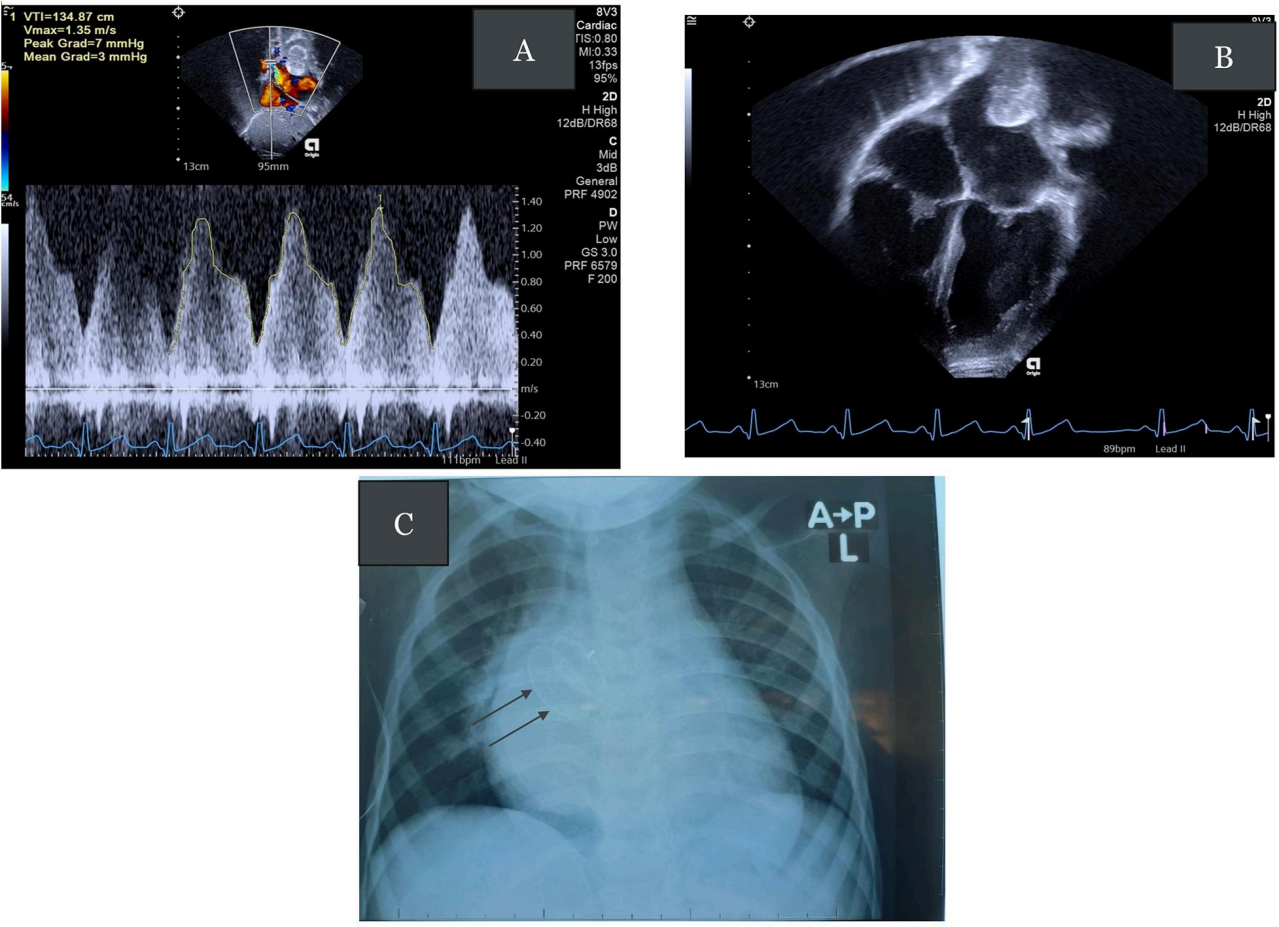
(A) Continuous wave Doppler tracing showing mild flow acceleration in the RUPV with a mean pressure gradient (PG) of 3 mmHg. (B) Apical four-chamber view showing the device posterosuperior to the LA. (C) Post-procedure chest radiograph showing satisfactory device position (black arrows).
